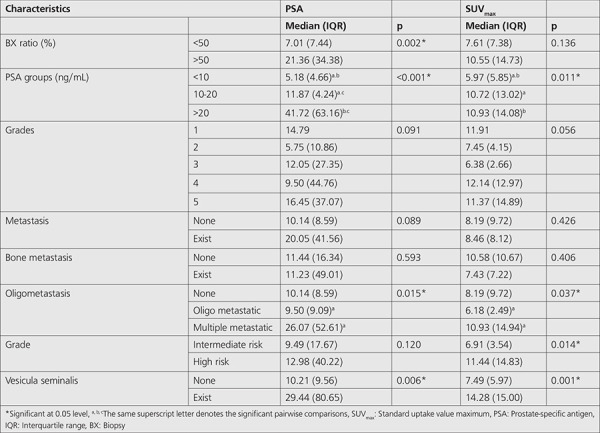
SUV_max_ and PSA according to some characteristics of patients
